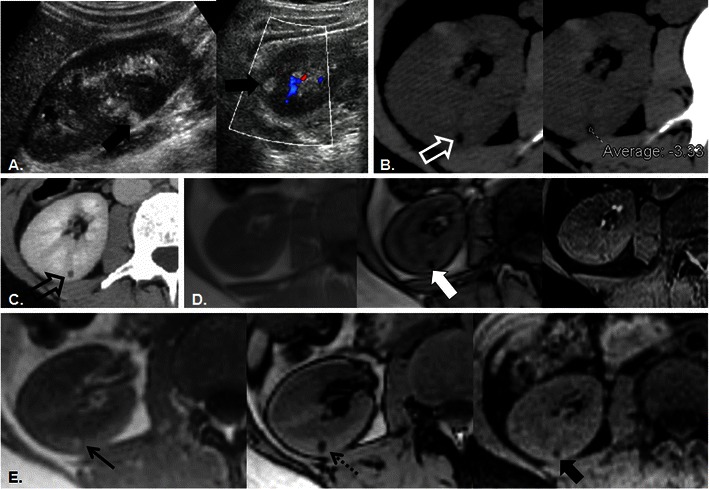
A 36-year-old man with incidental echogenic renal cortical nodule. Sagittal and transverse grey scale and colour (a) images of the right kidney depict a homogeneously hyperechoic nodule in the interpolar region (*white arrows*) which measures 8 × 6 × 6 mm in size. Axial unenhanced CT image (b) confirms the presence of a low-density nodule (*open white arrow*) with an ROI measurement of −3 HU. Pixel mapping was not performed and a follow-up CT was recommended. Six-month follow-up contrast-enhanced axial (c) CT image re-demonstrates the small renal cortical nodule (*open white arrow*), which is not changed in size but remains indeterminate; MRI was recommended. Axial T1-weighted IP, OP, and FS GRE images (*left to right* in d) reveal the renal nodule as a punctate focus of signal loss on OP image only (*white arrow*). The lesion was again considered indeterminate and follow-up was recommended. Axial T1-weighted IP, OP, and FS GRE images (*left to right* in e) performed 3 years later confirm the diagnosis of renal AML with persistent signal loss on the OP image (*dotted arrow*) and loss of signal intensity now noted on FS (*thick black arrow*) compared with IP (*thin black arrow*) T1-weighted images. In retrospect, the diagnosis was apparent on initial MRI examination (d); signal loss on OP imaging in uniformly echogenic nodules measuring <1 cm in size is confirmatory of AML
